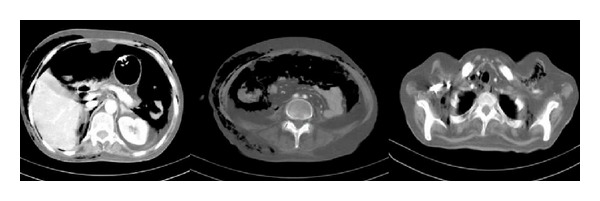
Thoracoabdominal CT, showing detached gastric wall from catheter insertion site and massive subcutaneous emphysema through cervical, thoracic, and abdominal region.
